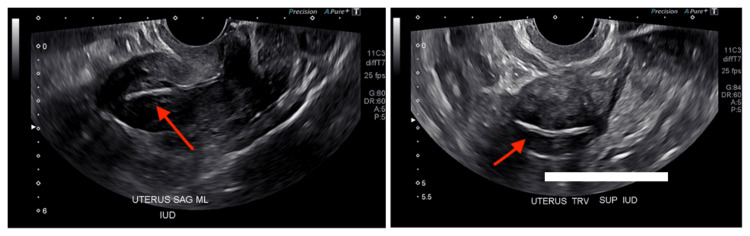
Sagittal and transverse transvaginal ultrasound images revealing the presence of the retained intrauterine device
